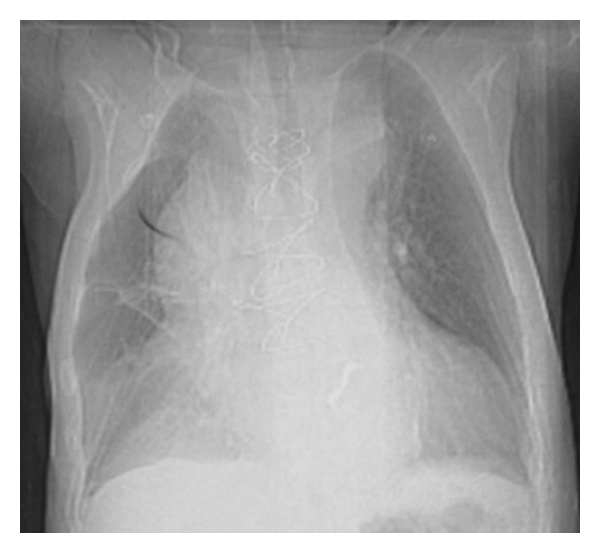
Chest X-ray revealed enlargement of mediastinum.
